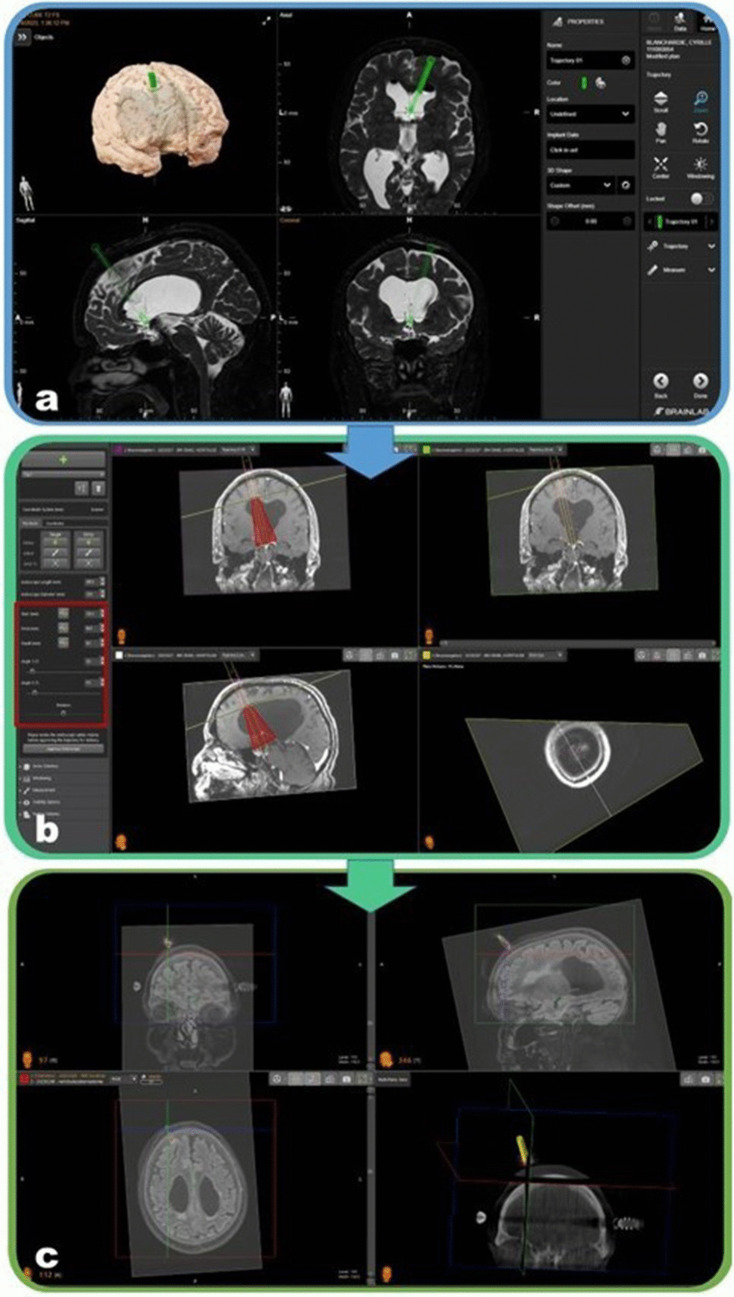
The preoperative planning of endoscopic third ventriculocisternostomy trajectory. a Using the BrainLAB Elements software, tridimensional gadolinium-T1- and T2-weighted sequences were used to define the most suitable trajectory defined as the shortest perpendicular pathway from right frontal gyrus to the interventricular foramen of Monro avoiding cerebral sulci and vessels. b The coordinates of the trajectory are transferred to the NeuroInspire software and endoscopic parameters are added: endoscopic outer diameter is set at 6 mm, while the endoscopic length is 150 mm. At this point, the workspace and the volume of movement of endoscope are defined (*red triangles*): the pivot point is placed at the cortico-ventricular junction, the depth is placed just below the floor of the third ventricle, and the degrees of freedom in the two axes (antero-posterior and latero-later angle) are chosen according to anatomical characteristics (*red square*). c An intraoperative tridimensional scan is acquired with cone beam O-Arm and co-registered with preoperative MRI through the Neuroinspire software to check the correct location of the robot along the axis of the planned endoscopic third ventriculocisternostomy trajectory using a Kirschner wire
